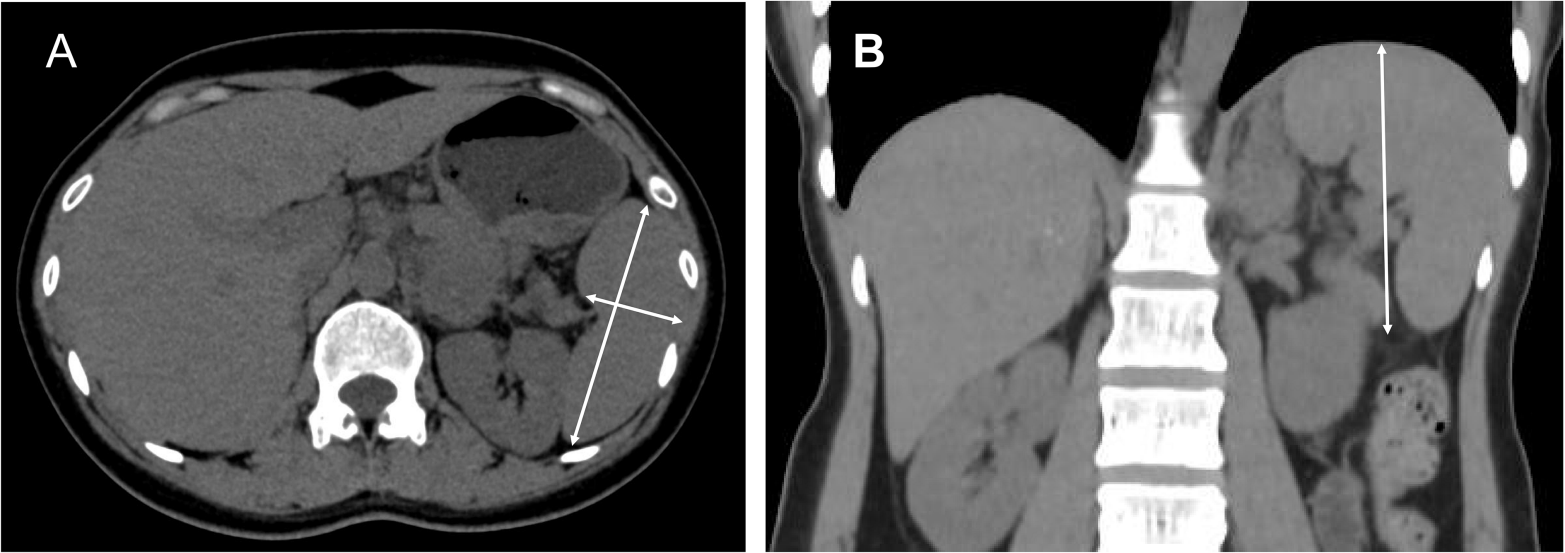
CT-based splenic volume measurement methodology. (A) Maximum splenic width (red line) and hilum thickness (blue line), measured perpendicular to the splenic width plane passing through the hilum. (B) Splenic height (yellow line), defined as the longest craniocaudal dimension.
